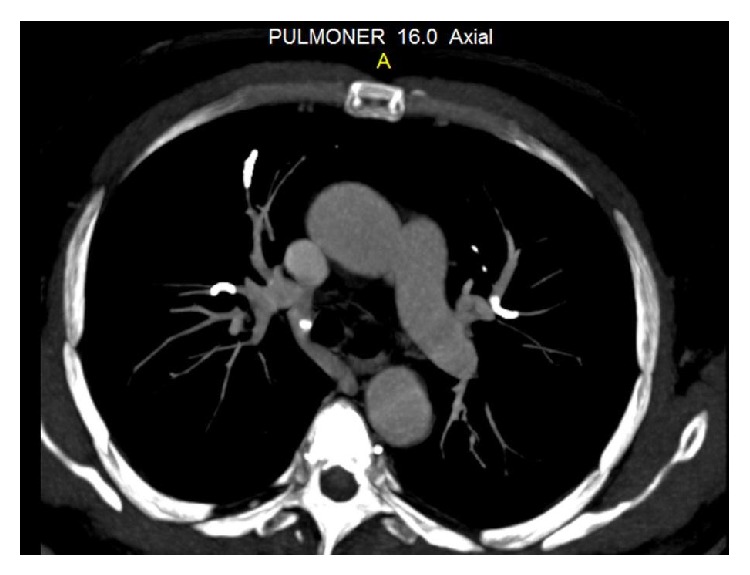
Axial view of thorax CT showing multiple, linear hyperdensities in bilateral lobar pulmonary artery branches.
